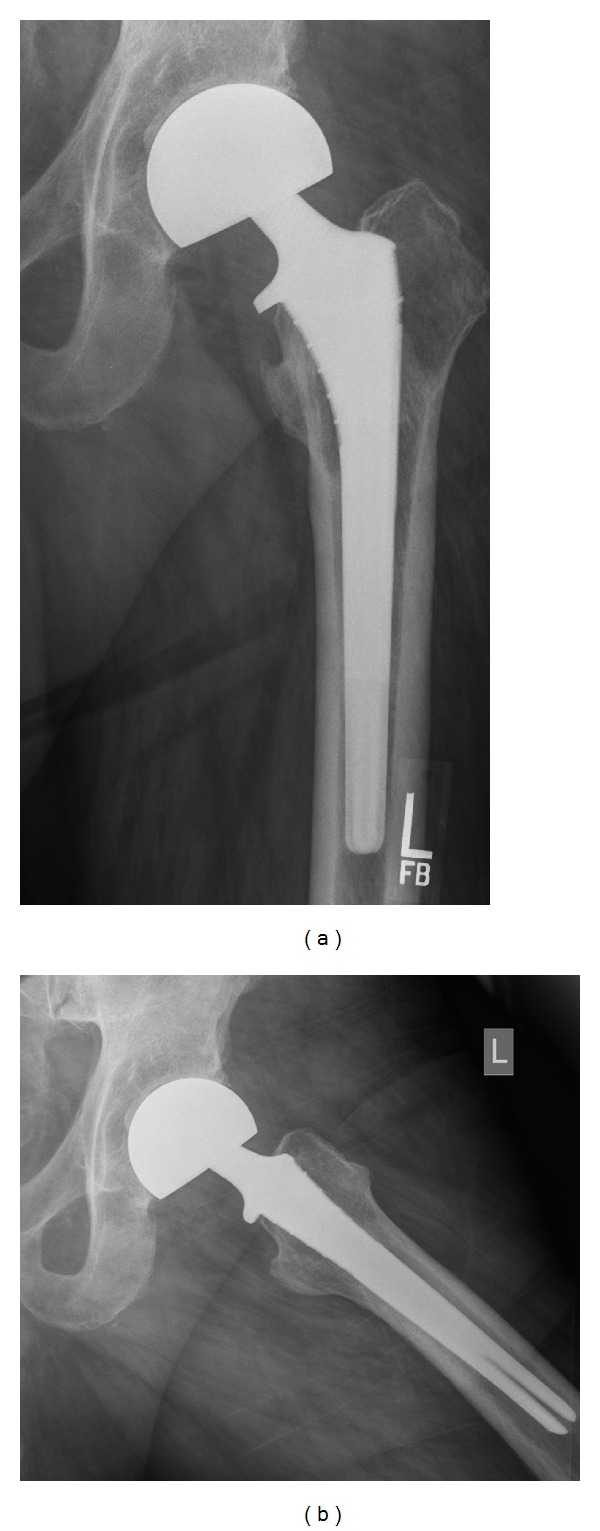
Same patient—AP and lateral radiographs—15 years following hemiarthroplasty.
